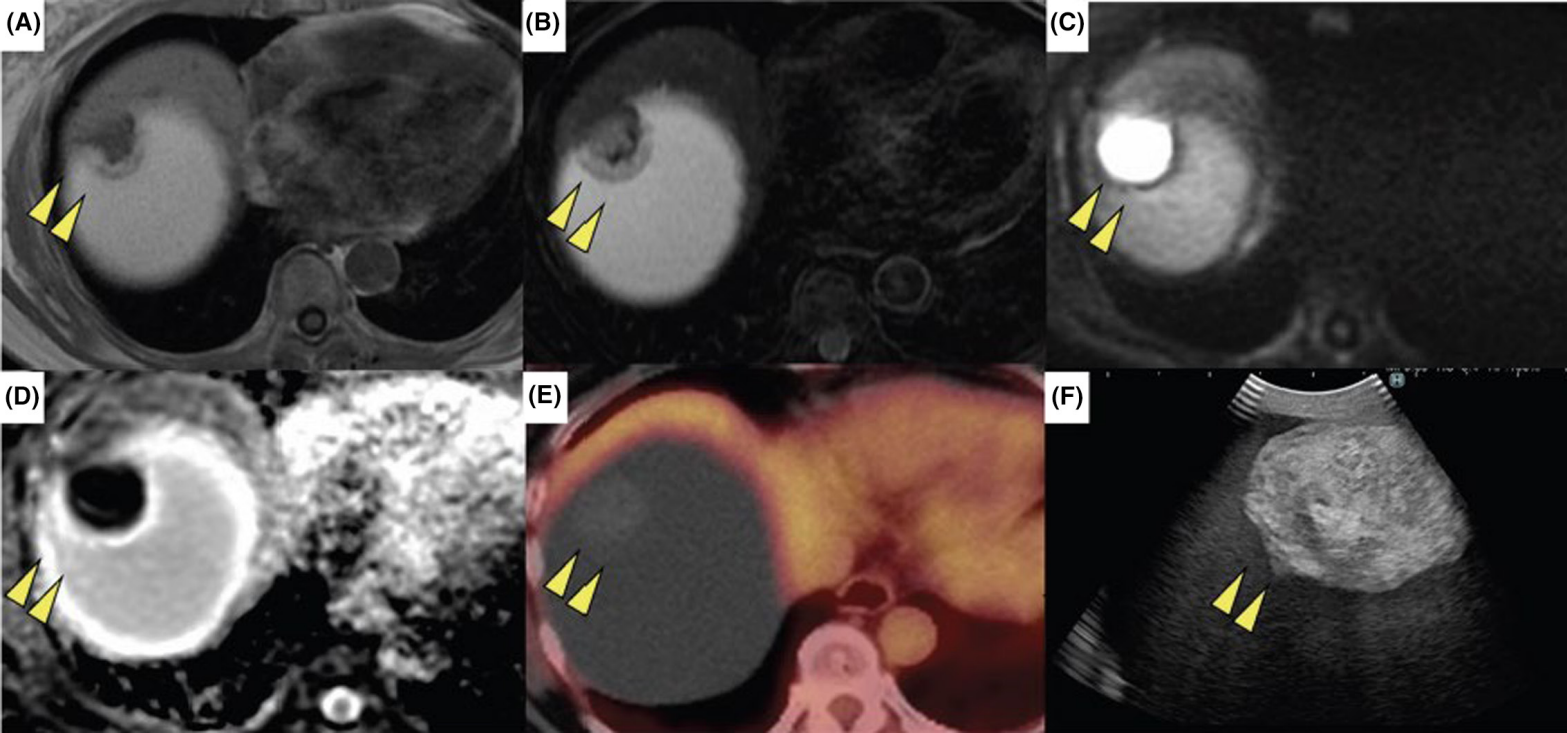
Preoperative magnetic resonance imaging (MRI) and positron emission topography (PET). (A) T1 weighted MRI scan shows a low signal intensity in the nodule within the liver cyst. (B) T2 weighted MRI scan shows an intermediate signal intensity in the nodule. (C) Diffusion‐weighted MRI scan reveals a high signal intensity in the tumor. (D) Apparent diffusion coefficient map MRI showing low signal intensity in the tumor. (E) PET shows no obvious hyperaccumulation of fluorodeoxyglucose in the nodule. (F) Intraoperative ultrasonography reveals uniform high echoic lesion with clear boundary.
